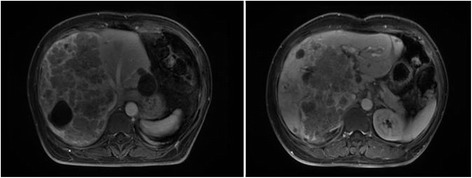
MRI imaging of the mass revealed tumor distributed at the right hepatic trisection, adjacent to the left branch of portal vein at primary diagnosis
